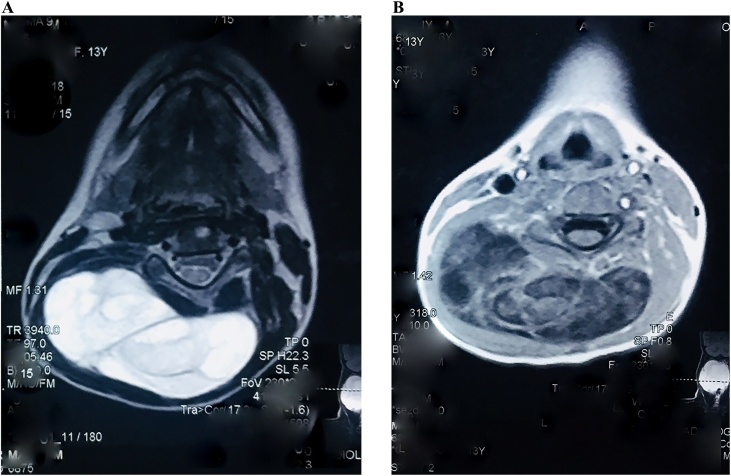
MRI neck T1 and T2 axial images show a multilocular cystic lesion.
